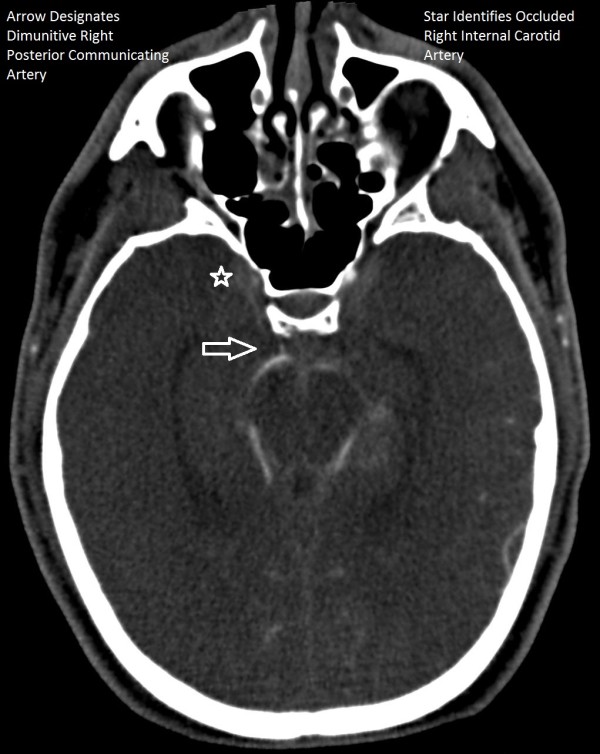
CTA brain coronal image demonstrating diminutive right posterior communicating artery.
